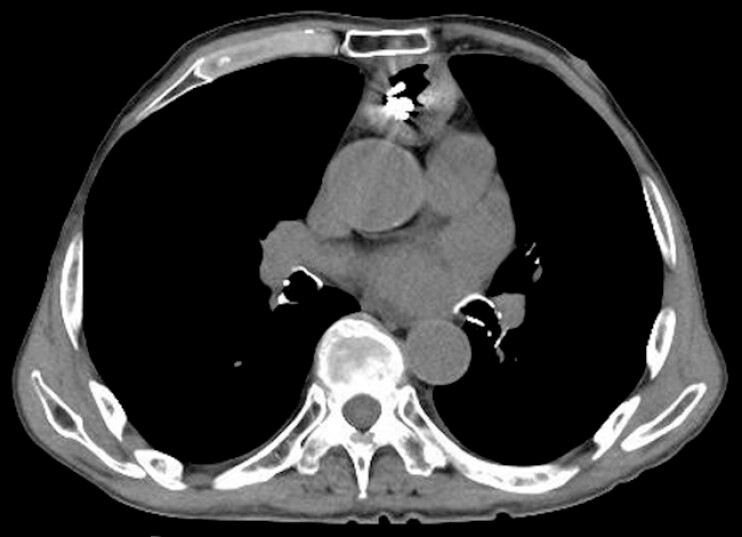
CT showing proximity of the sutured site to the ascending aorta. CT, computed tomography.
